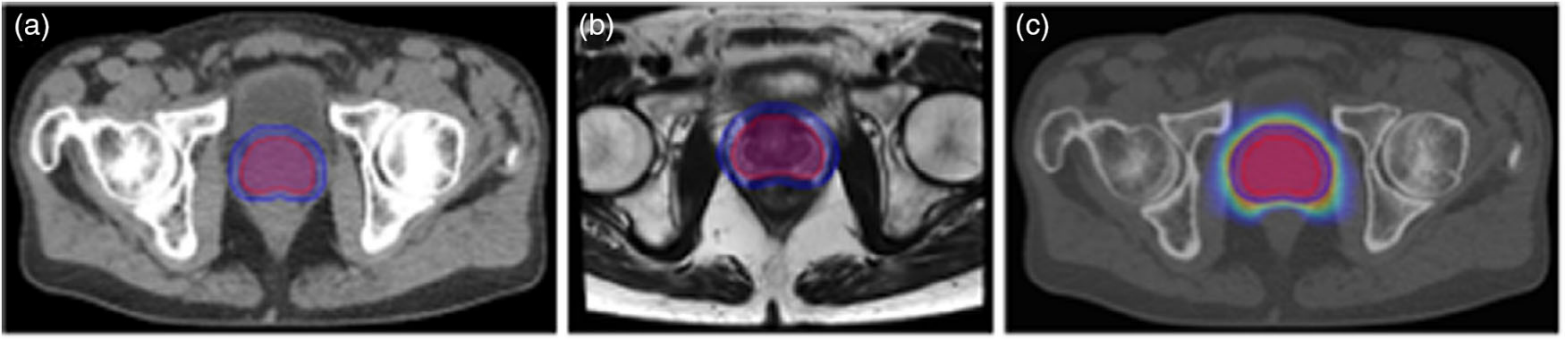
Imaging of a 65‐year‐old male with intermediate‐risk (Stage T1c) adenocarcinoma of the prostate procured from our institution. (a) Axial view of the planning CT with GTV and PTV outlined. (b) Axial T2‐weighted MRI showing GTV and PTV. (c) Axial view of the planning CT with GTV and PTV contours, plus a dose color wash; dose window level from 35 to 70 Gy.
